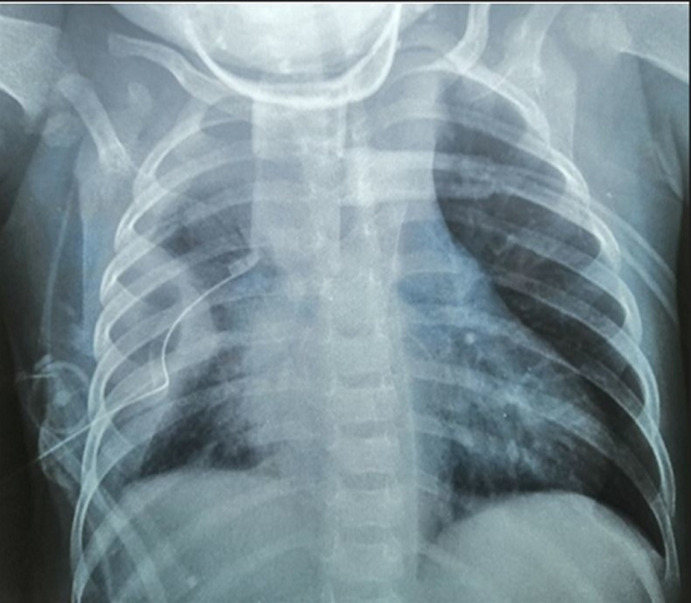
the immediate chest X-ray control showing a mediastinal enlargement and a right septate pleurisy with a drain well-placed
